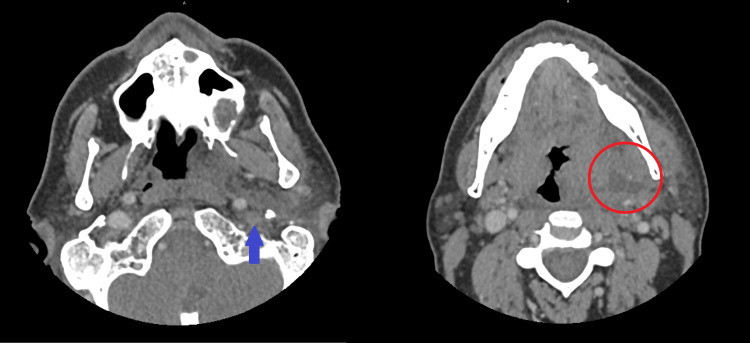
CT showing left peritonsillar abscess (red circle) and left internal jugular vein thrombosis (blue arrow) CT: computed tomography
